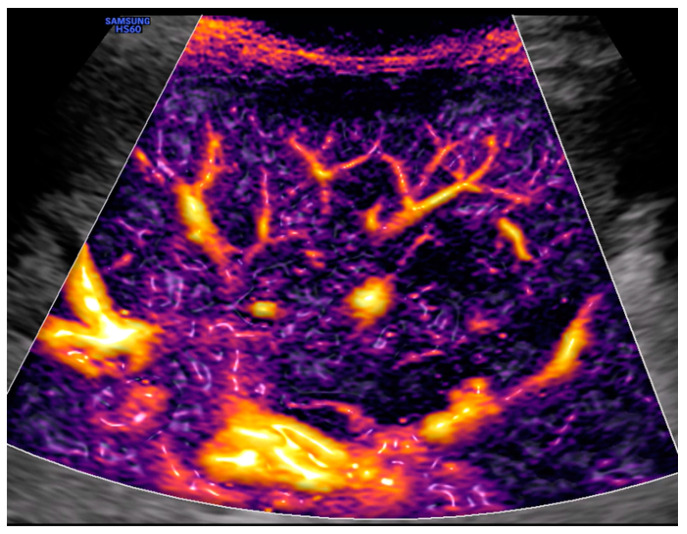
Left upper quadrant view: spleen and Doppler with M-Flow technology. Image courtesy of J. Osterwalder.
